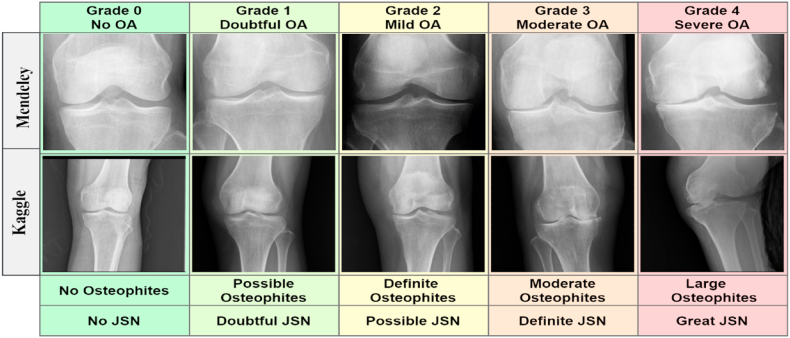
X-ray images of the five grades from two datasets.
